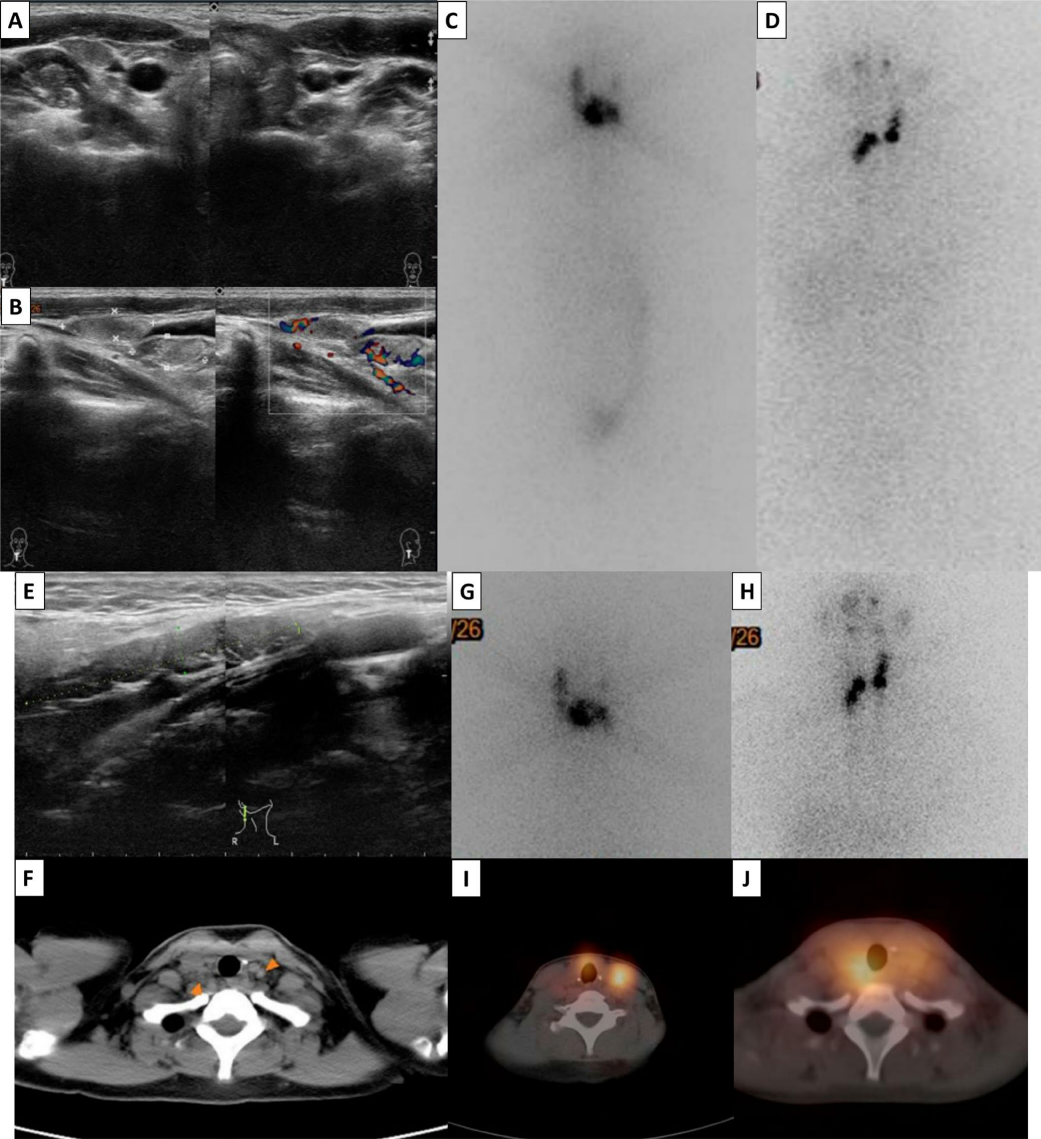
A case with persistent disease. An 11‐year‐old prepubertal girl with papillary thyroid cancer underwent total thyroidectomy and was transferred to our hospital. (A and B) Ultrasonography showed no lesions in the postoperative thyroid bed, but there were massive cervical lymph node metastases on both sides. (C and D) An RAI dose of 120 mCi was administered. Post‐RAI imaging showed good iodine avidity in the right cervical metastatic lymph nodes. (E and F) During the follow‐up the next year, cervical metastatic lymph node progression was noted in ultrasonography and a computed tomography scan. (G–J) After bilateral neck lymph node dissection, the patient received another RAI dose of 150 mCi. The residual left lower cervical lymph node avidity was found on post‐RAI imaging.
